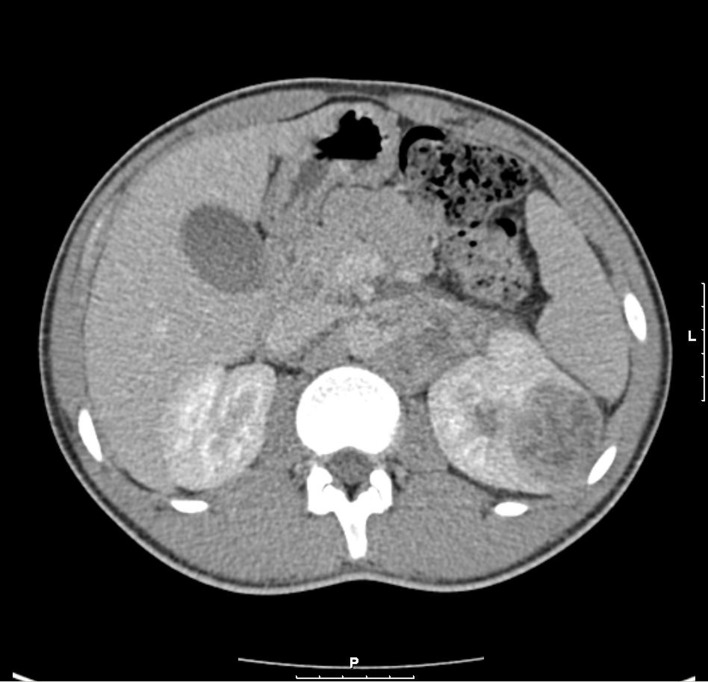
CT shows left renal enhancing lesion with para-aortic lymph node mass.
